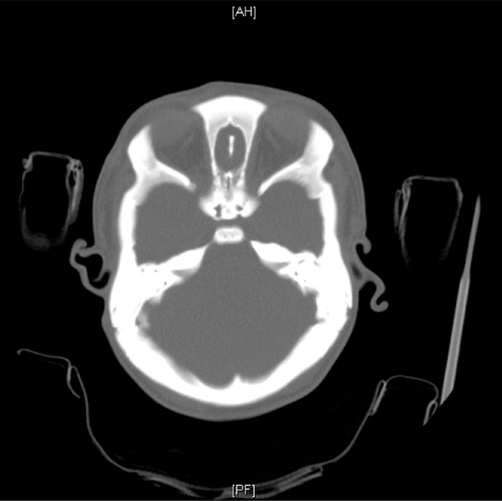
CT head (3-D reconstruction) showing oxycephaly with bone growth around an open fontanelle and hypertrophy of the metopic suture posteriorly and the coronal sutures medially.
